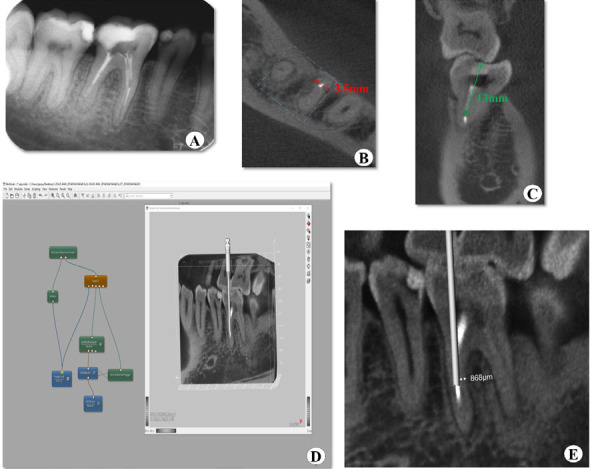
(A) Preoperative radiograph showing the broken instrument in the mesial canal. (B) Crossectional view of CBCT confirming broken instrument in the mesiobuccal root canal of tooth #30 and 3.5mm mesiodistal distance (red arrow). (C)Coronal view of the CBCT image indicating the distance between the open access and the coronal end of broken instrument (green arrow)=13mm. (D) Placement of a virtual micro-trepan bur around the broken instrument using Mevislab. (E) Remaining dentin thickness=868μm obtained.
